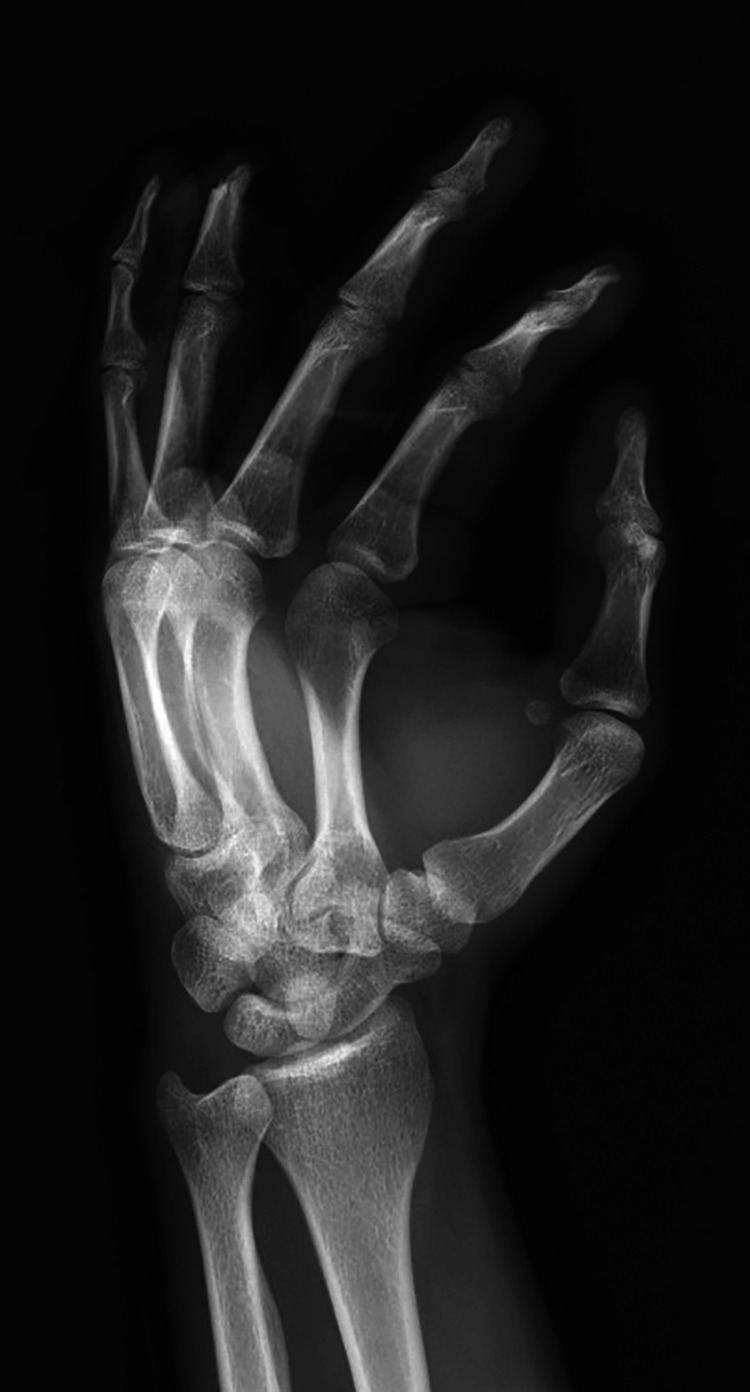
Oblique plain radiograph of the left hand demonstrating amputation through the ring finger middle phalanx.
